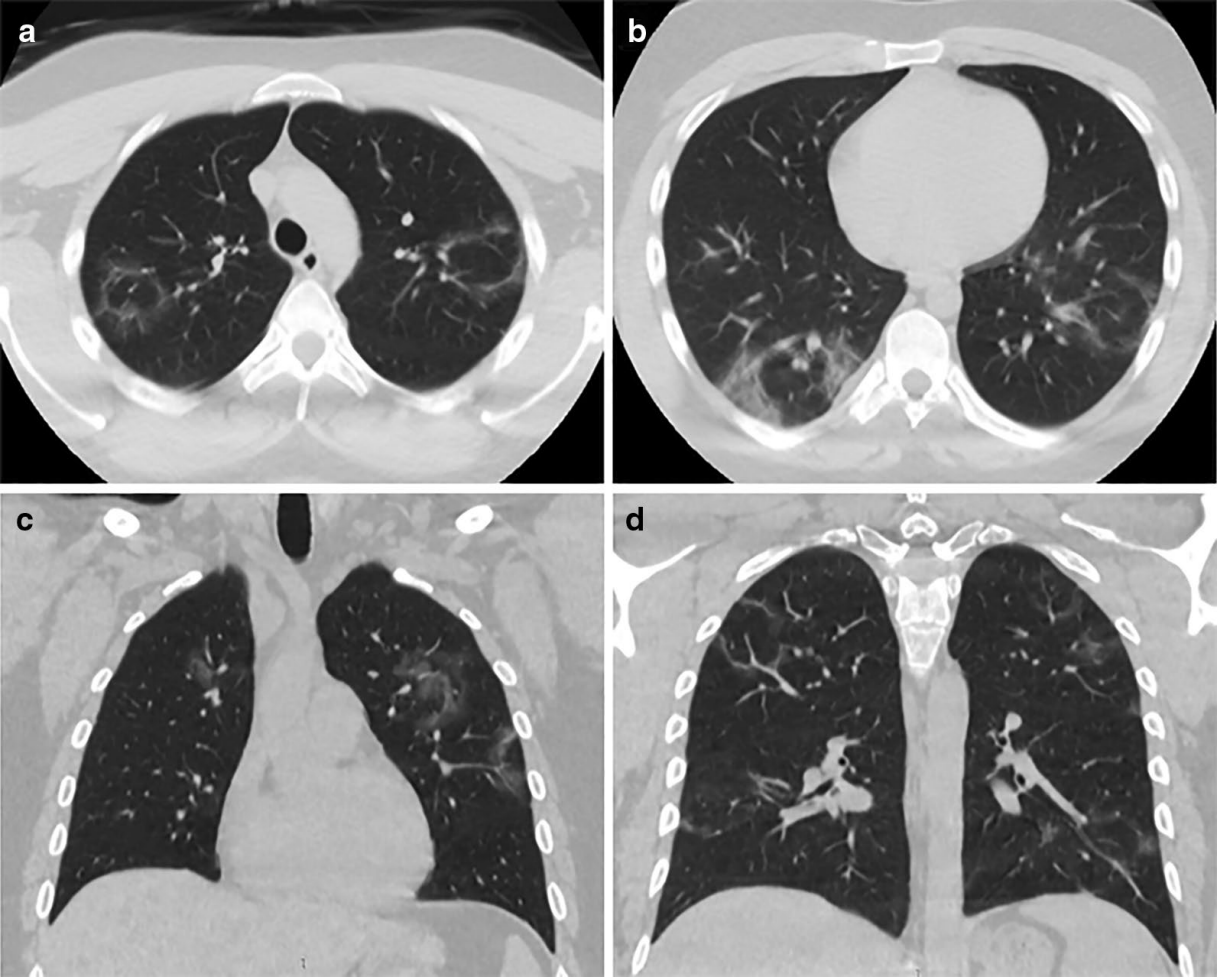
A 28-year-old man with positive RT-PCR findings for COVID-19. a–d Noncontrast axial CT images of the chest show bilateral peripheral ground-glass opacities, bilateral reverse halo sign, and prominent vessel inside. This is in keeping with his CO-RADS 5 and typical RSNA classification
